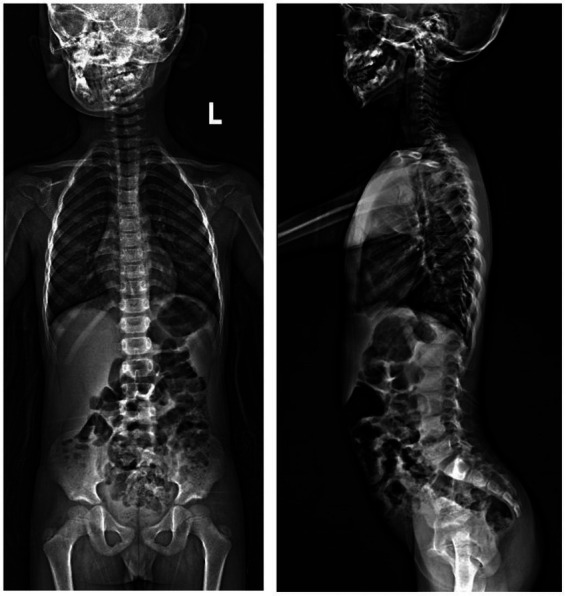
X-ray photographs showed no significant abnormalities of skeleton.
